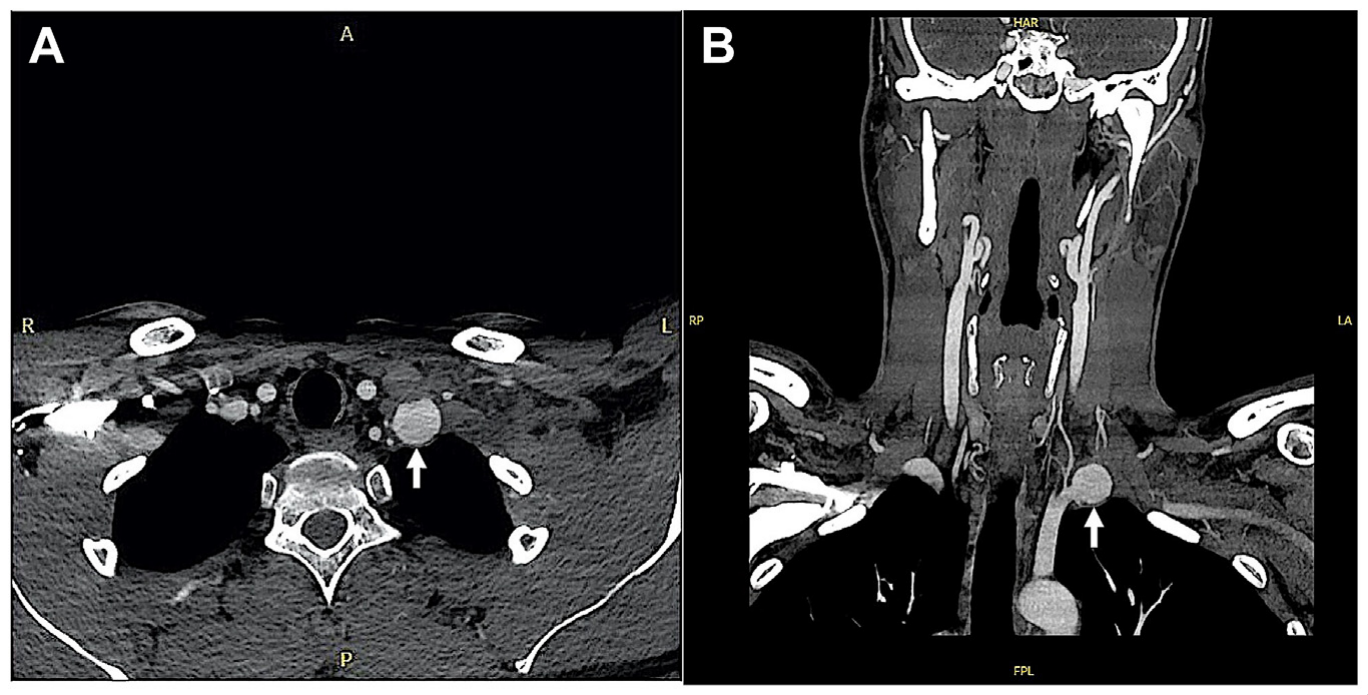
a, Axial view of computed tomography angiogram showing a 1.9 × 1.5-cm subclavian artery mycotic aneurysm (SAMA; *arrow*). b, Coronal view of computed tomography angiogram showing a 1.9 × 1.5-cm SAMA (*arrow*).
